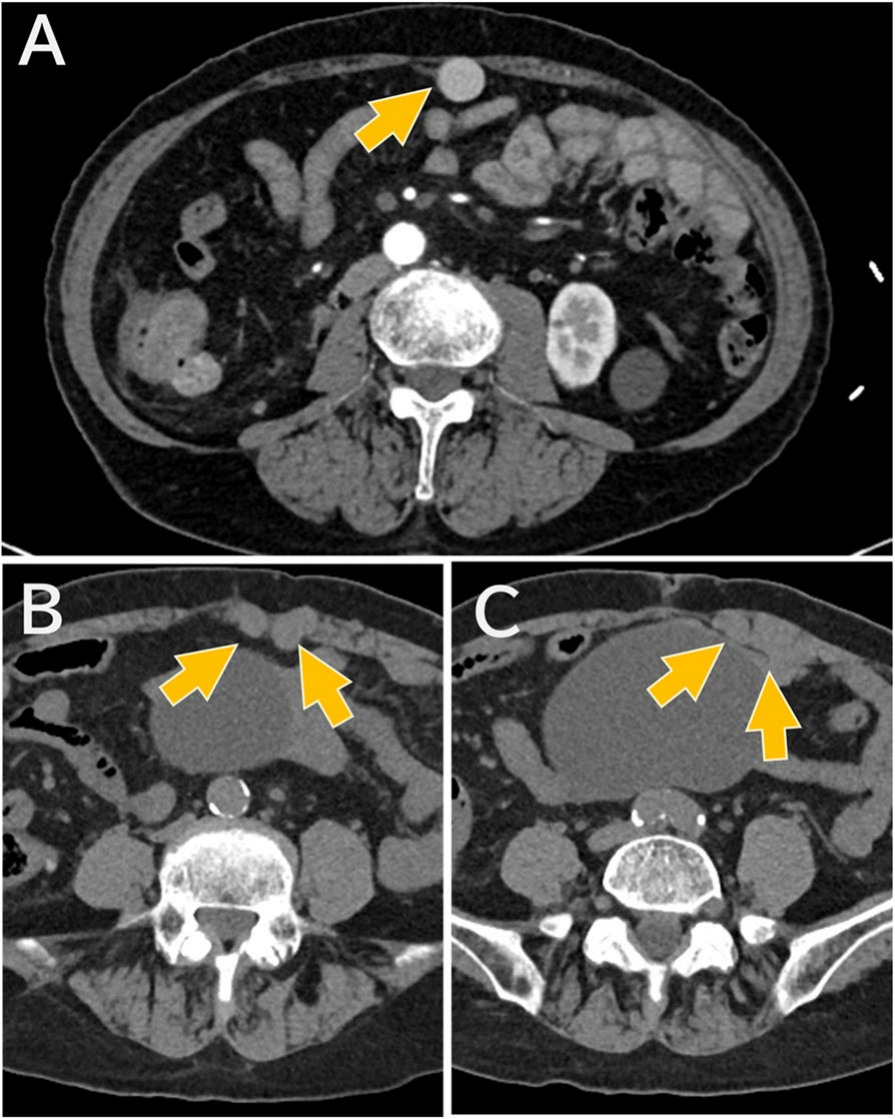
Preoperative CT images showing dilated abdominal wall veins. (A) Enhanced CT image showing a prominent collateral vein (arrows) near the abdominal wall. (B, C) Unenhanced CT images at progressively caudal levels, demonstrating the course of the dilated vein (arrows) as it runs through the rectus abdominis muscle.※Contrast-enhanced images are not available for these slices
